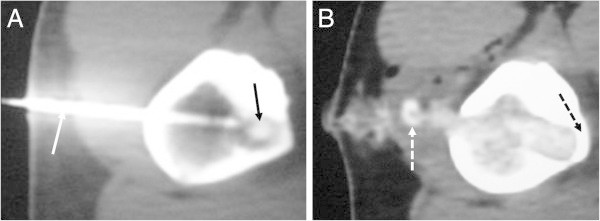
A. CT showing the well positioned K-wire (white arrow). Access to the nidus (black arrow) via the opposite cortex was useful in this case to avoid the neurovascular bundle. B. CT after resection. Note that the cortex was not totally drilled. The black dashed arrow indicates the remaining cortex. Small bone debris was left in the path and is considered harmless (dashed white arrow).
